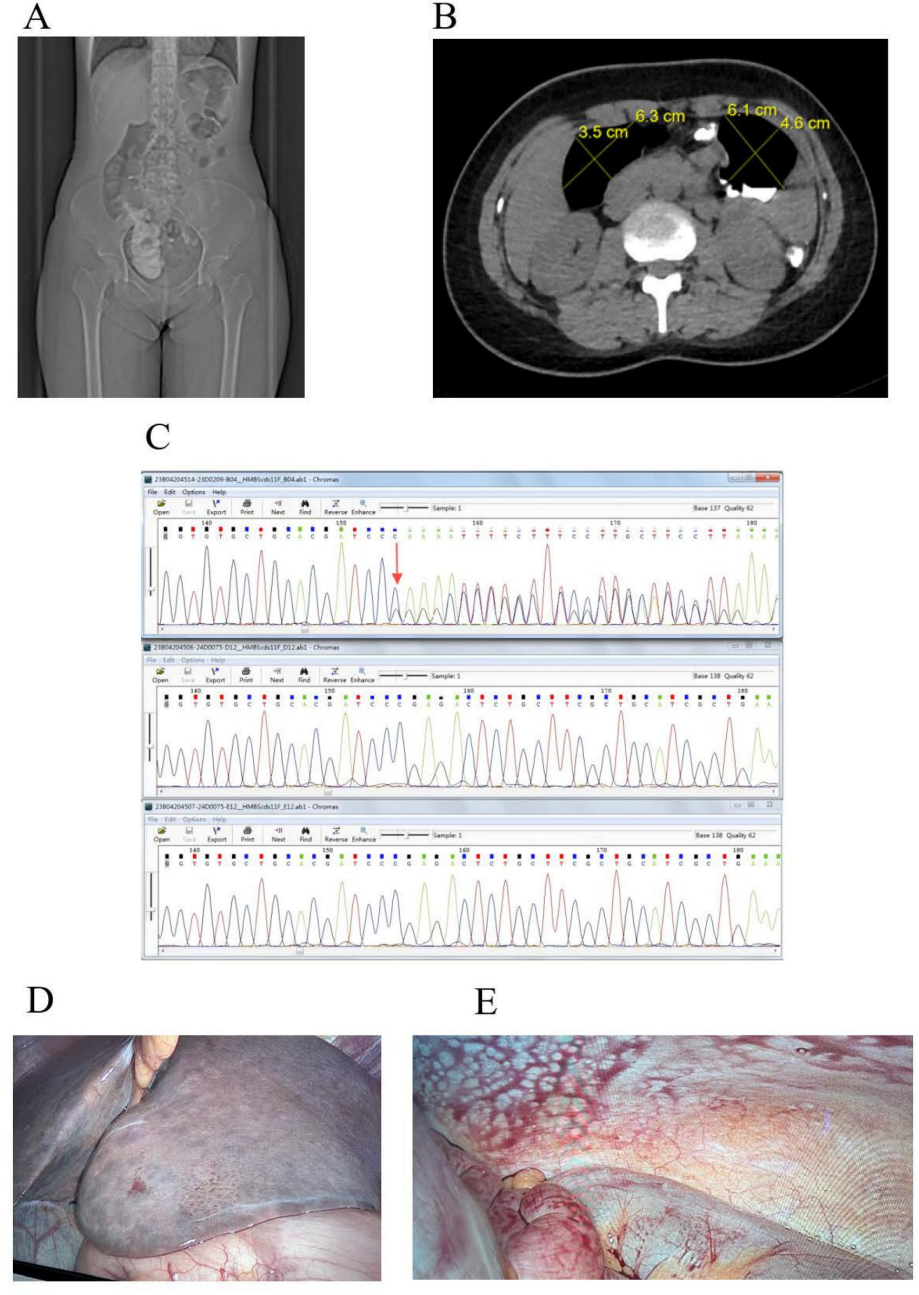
Imaging, genetic, and intraoperative features of acute intermittent porphyria complicated by megacolon. (A) Gastrointestinal contrast studies showing dilation of the right colon; (B) Preoperative CT scan demonstrating dilation of the right colon; (C) Sanger sequencing chromatograms of HMBS exon 11. Upper panel: index patient (proband) showing heterozygous c.723delC (p.Glu242Argfs*13). Middle panel: mother, wild-type sequence. Lower panel: father, wild-type sequence; (D) Intraoperative laparoscopic view showing a congested liver; (E): Congested bowel observed during surgery. AIP, acute intermittent porphyria; CT, computed tomography.
